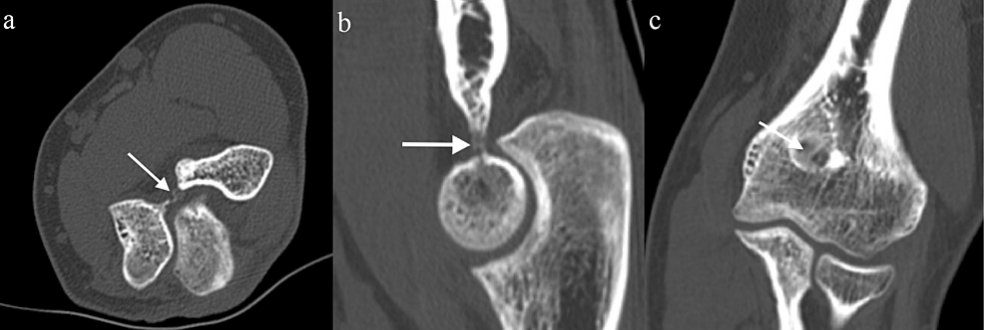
Preoperative CT scan of the left elbow Axial (a) and sagittal (b) images show a sub-centimeter lucent lesion with central sclerotic focus (arrow) within the bony margin of the olecranon fossa. A small amount of adjacent sclerotic reactive bone is seen on the coronal (c) images (arrow).
